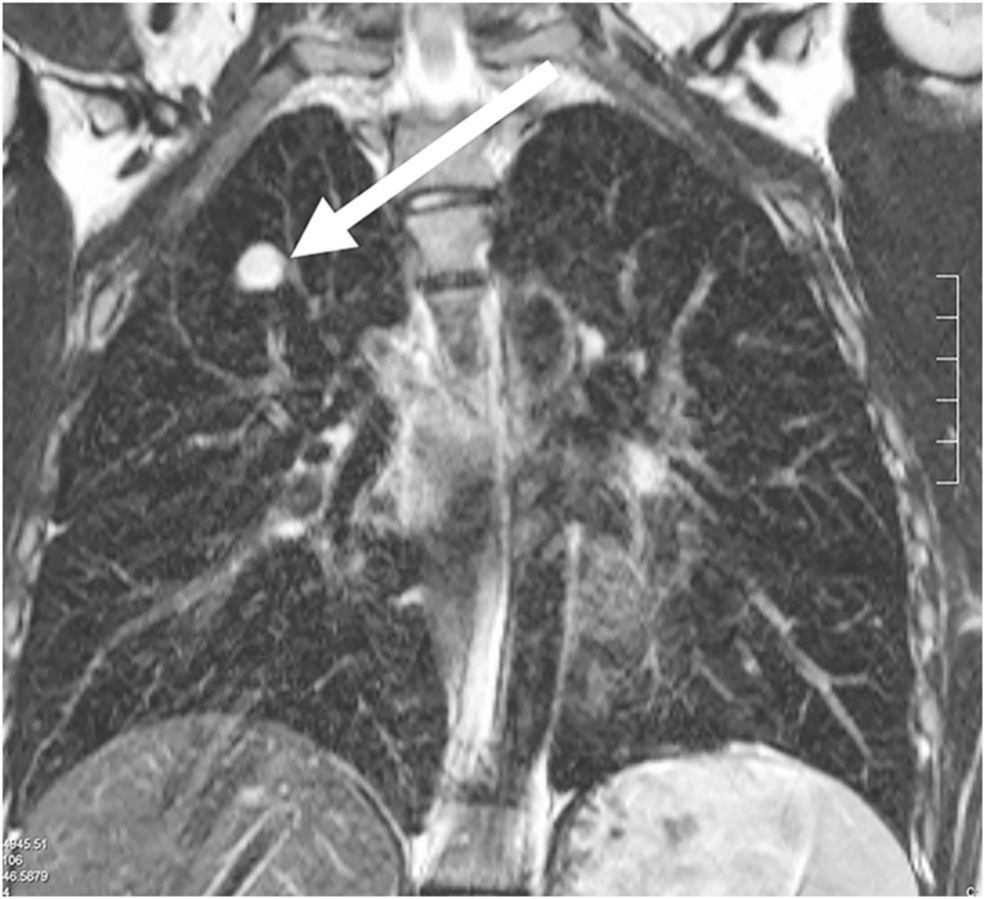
Hamartoma on lung MR in a 16-year-old girl (coronal T2-weighted-turbo spin echo, MR parameters in Table 2). Image shows a hamartoma (*arrow*) in the right upper lobe. The lesion was an incidental finding on a chest radiograph taken because of a cough. On MR, the nodule presents homogeneous hyperintensity
